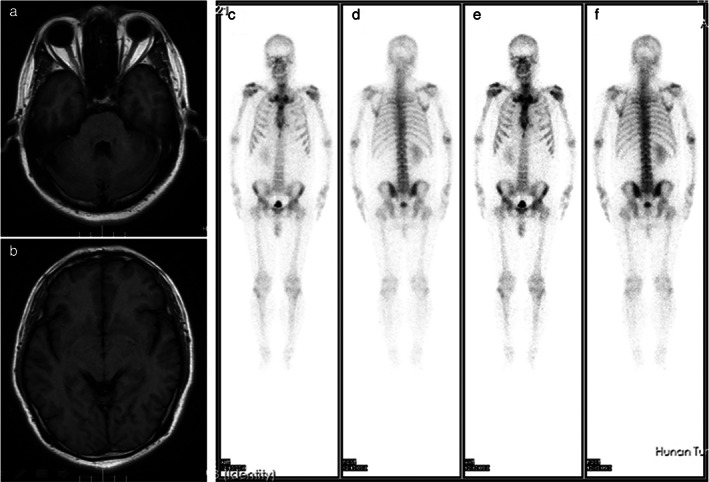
No abnormalities were reported with bone single‐photon emission computed tomography or brain magnetic resonance imaging
